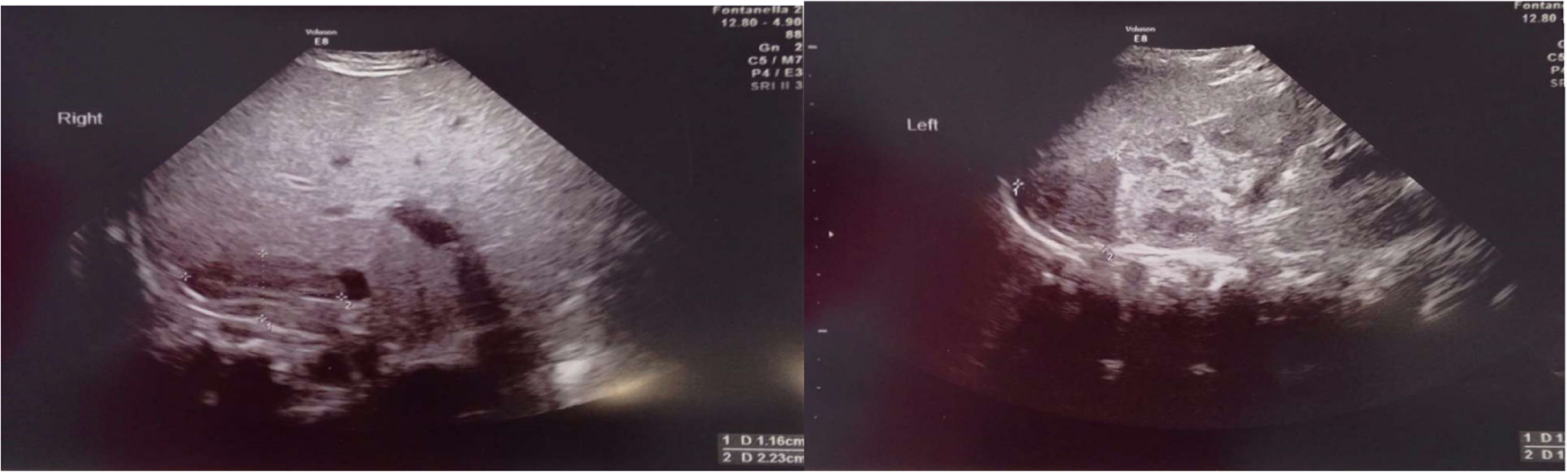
Ultrasound changes of NAH in follow-up with modification of the ultrasonographic appearance
